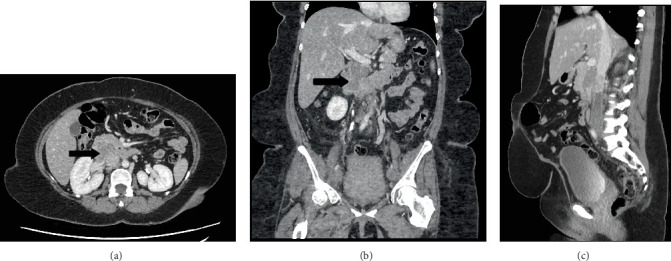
CT abdomen. (a) Axial, (b) coronal and (c) sagittal scans in portal venous phase show a heterogeneous mass (black arrow) within the region of the pancreatic head.
